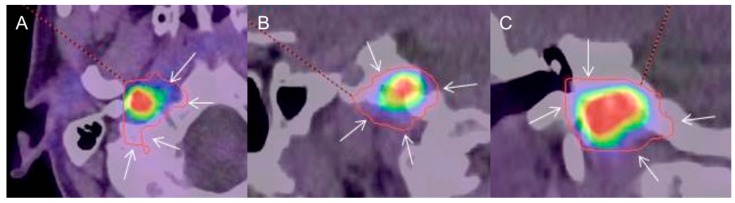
Typical example of gross tumor volume assessed by tumor-to-brain ratio (GTV_TBR_) (red contour) evaluated on ^18^F-dihydroxyphenylalanine (^18^F-FDOPA) combined positron emission tomography/computed tomography (PET/CT) images (A: axial, B: sagittal, C: coronal) in a patient with a sporadic right skull base paraganglioma (SBPGL). A threshold value of 1.6 over the background uptake was used as the reference for semi-automatic definition of GTV. Note that GTV_TBR_ largely exceeds the metabolic tumoral edges (arrows).
